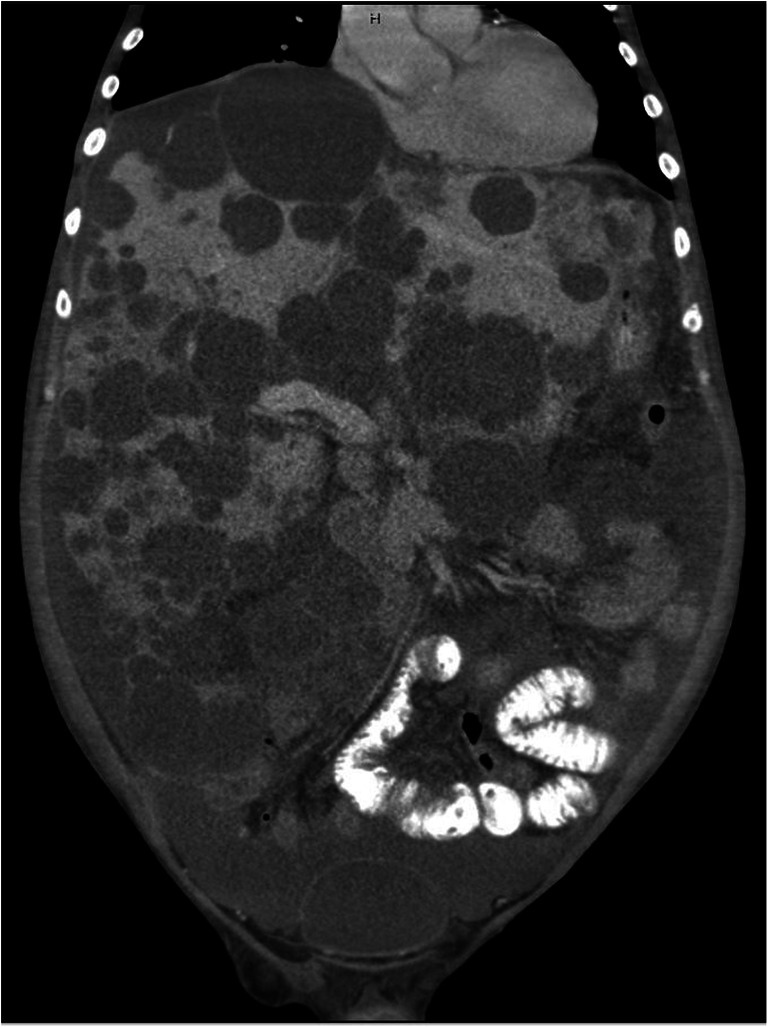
Coronal presentation of the portal vein in a polycystic liver. The kidneys are also polycystically degenerated as seen in the CT scan (CT Revolution 256 slice, GE Healthcare, USA) before transplantation
